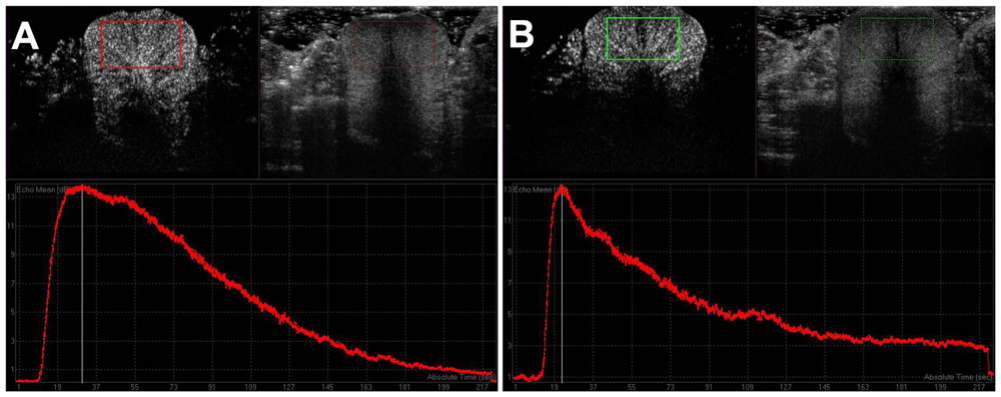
Analysis of contrast-enhanced ultrasound in the microbubble-enhanced ultrasound group. The upper panels in A (before treatment) and B (after treatment) showed the contrast-enhanced ultrasound image with two modes: contrast mode (left) and tissue mode (right). A rectangular region of interest was defined inside the frontal prostate, excluding the acoustically attenuated area. The lower panels showed a time intensity curve (TIC) generated automatically by QLAB software. (A) After a rapid ascension in acoustic intensity, the TIC showed a relative plateau around peak intensity (representing microbubble diffusion to the mesenchyme) and then a slow descent. (B) The ascension and descension of the TIC after treatment were steeper, and the diffusion plateau became imperceptible or even disappeared.
